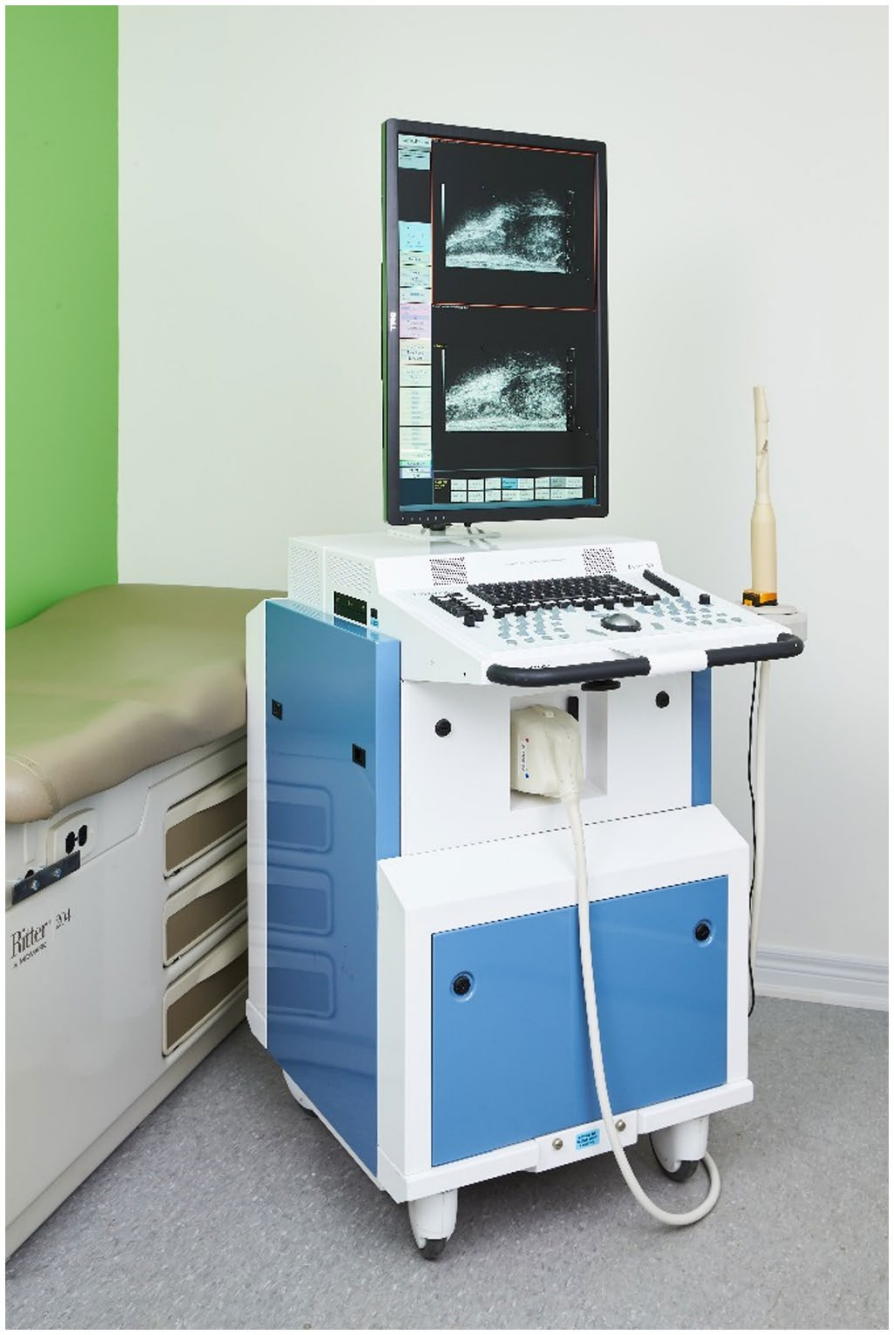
First‐generation ExactVu micro‐ultrasound system used in this study
